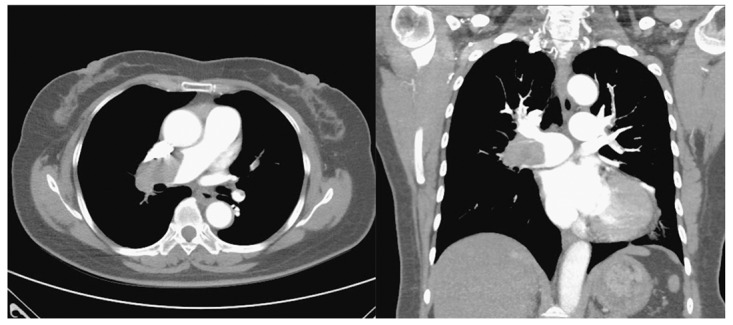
Computed tomography angiography demonstrates massive thrombosis in bilateral pulmonary arteries, predominantly right-sided.
